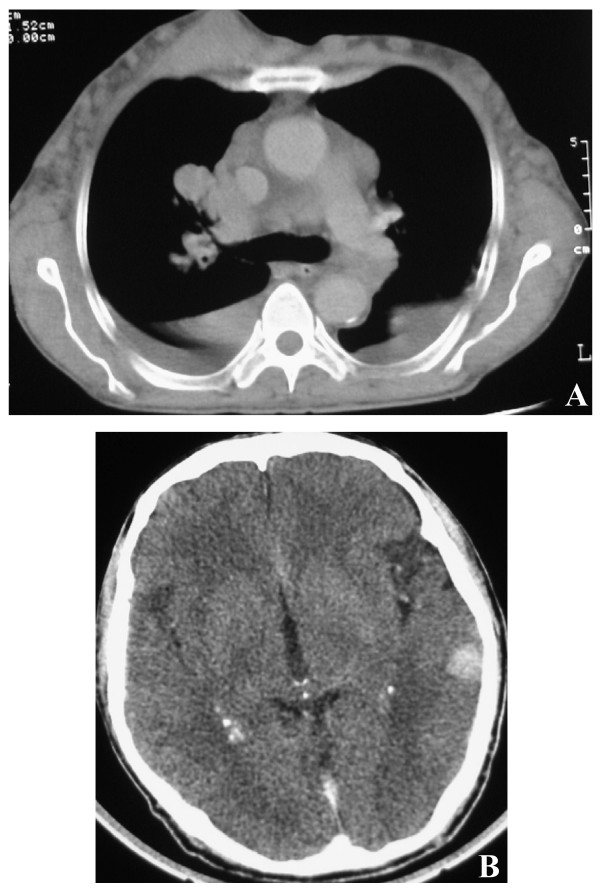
(A) Computed tomography image shows a lobulated, irregularly shaped mass 7 × 4.5 cm in diameter with central hypodensity in the right atrium. (B) Computed tomography image shows a brain metastasis.
